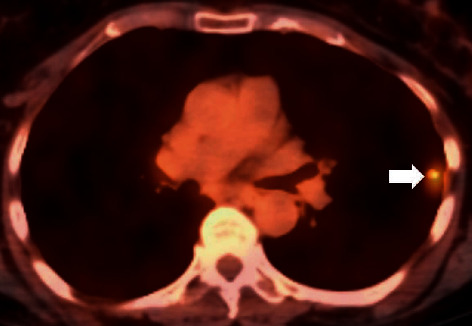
Axial, fused FDG PET–CT image shows increased FDG uptake in the left upper lobe nodule with SUV of 4.2 (arrow).
